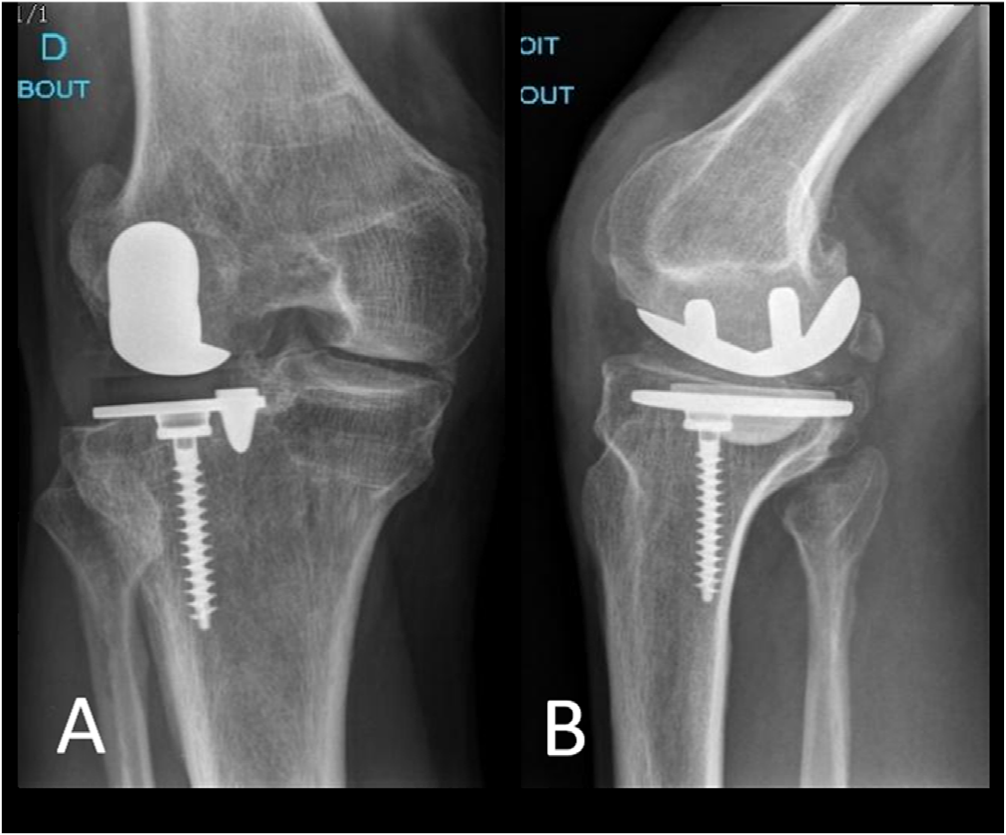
Post-operative plain radiographs, right knee: (A) anterior view, (B) lateral view.
